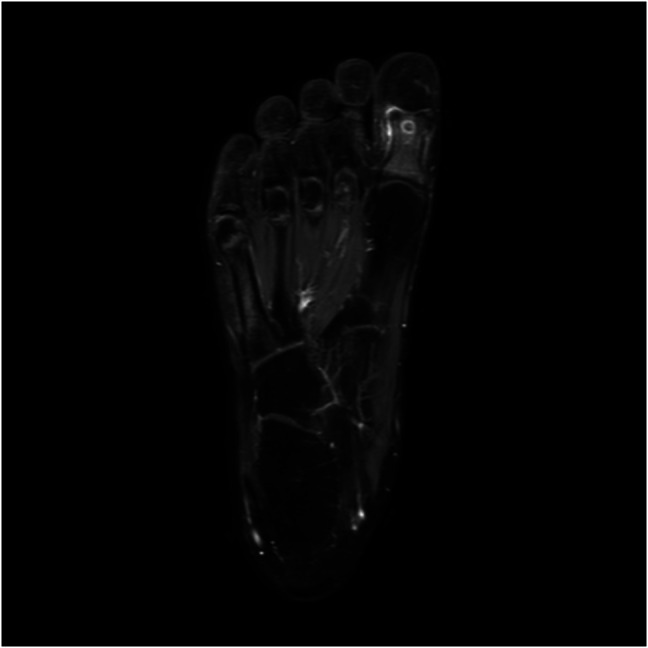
Gadolinium-enhanced MR T1-weighted axial images indicating a 4-mm bony lesion with high signal intensity in the periphery and low signal intensity at the center along with diffuse bone marrow edema and periosteal inflammatory changes in the proximal phalanx of the great toe.
